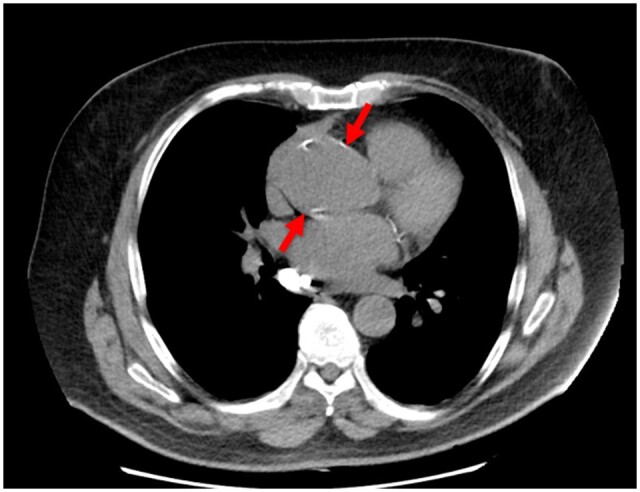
Axial view of missed findings for aortic dilatation (arrows) on a non-contrast CT chest.
